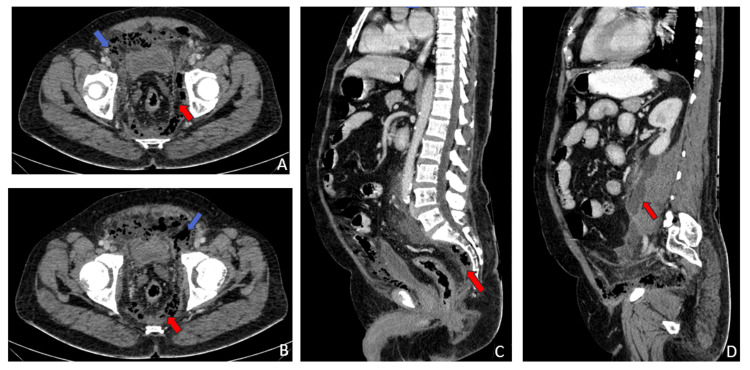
(A, B) Axial CECT of the abdomen and pelvis showing hypodense peripherally enhancing collections with multiple air foci in the presacral region (red arrows) in continuum with similar collections in the perivesical region and underneath the anterior abdominal wall (blue arrows). (C, D) Sagittal reformatted CECT images showing collection with air foci in the presacral region (C) with superior retroperitoneal extension and involving the left psoas muscle (D). CECT: contrast-enhanced computed tomography
